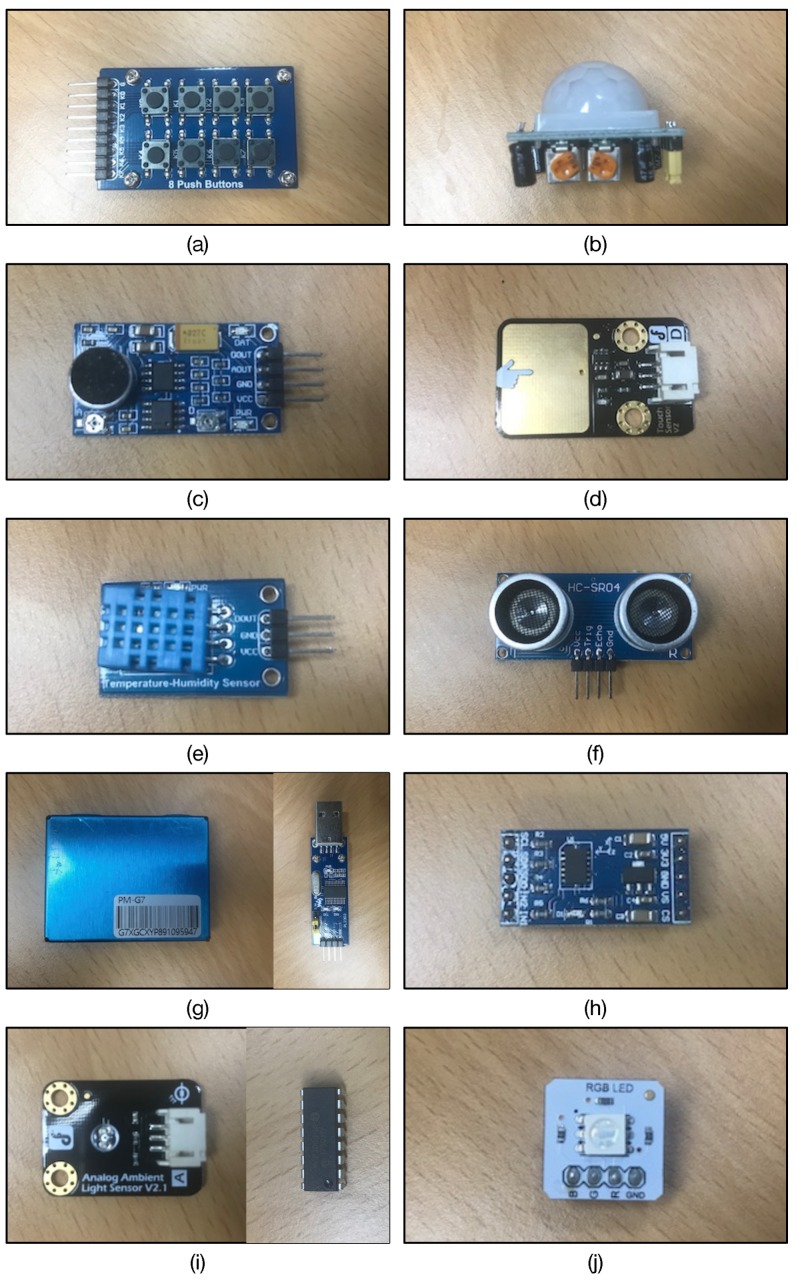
The photos of sensors and actuators deployed in our implementation work; (a) eight-pushbutton matrix, (b) passive infrared (PIR) sensor, (c) ambient sound sensor, (d) capacitive touch sensor, (e) temperature sensor, (f) ultrasonic sensor, (g) particulate matter sensor (left) with PL2303 USB-to-UART converter (right), (h) accelerometer, (i) ambient light sensor (left) with MCP3008 analog-to-digital converter (right), (j) RGB LED.
